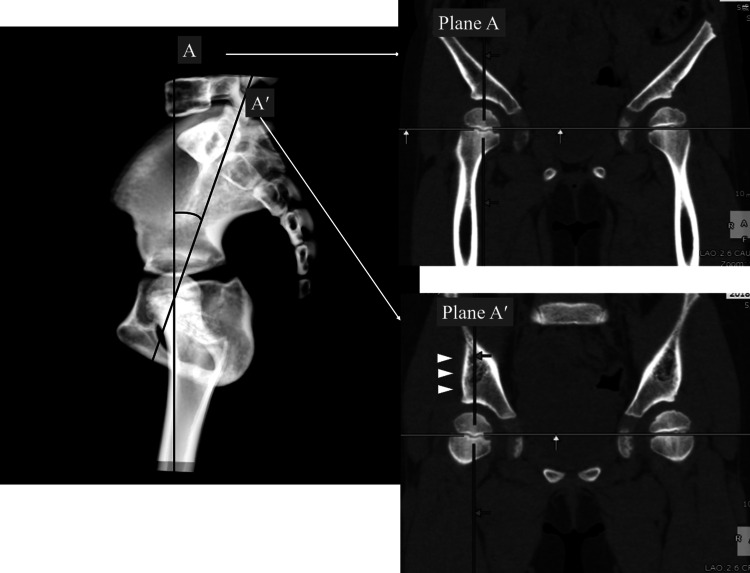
Standard plane on computed tomography The iliac contour is not parallel to the sagittal plane in plane A but is parallel to the sagittal plane in plane A′ (white allow heads). Angle AA′ in this figure is negative because plane A′ is obtained by rotating plane A clockwise, viewing from the left side.
